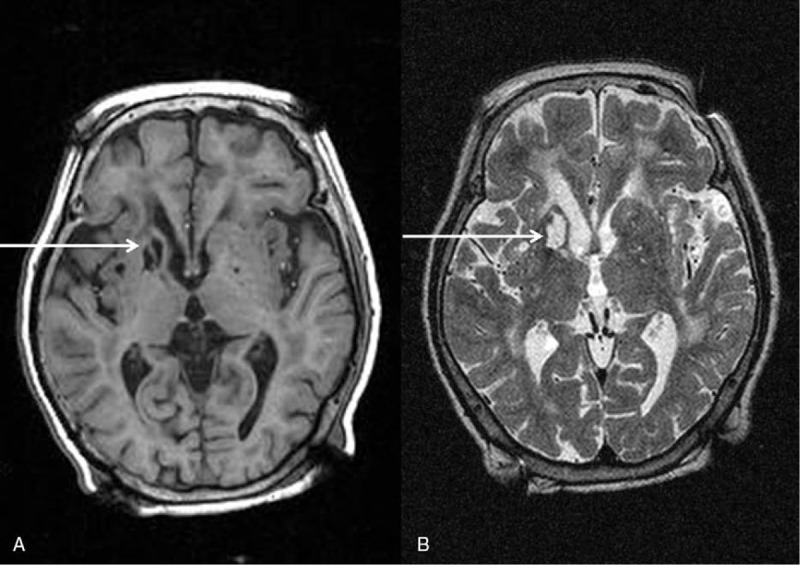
T1 (A) and T2 (B) signals from preoperative MRI showed changes associated with ischemic leukomalacia at the head of the right caudate nucleus (white arrow), which were regarded as sequalae of past cerebral ischemia. Electrode insertion into the ischemia-damaged brain carries a risk of further damage or hemorrhage.
